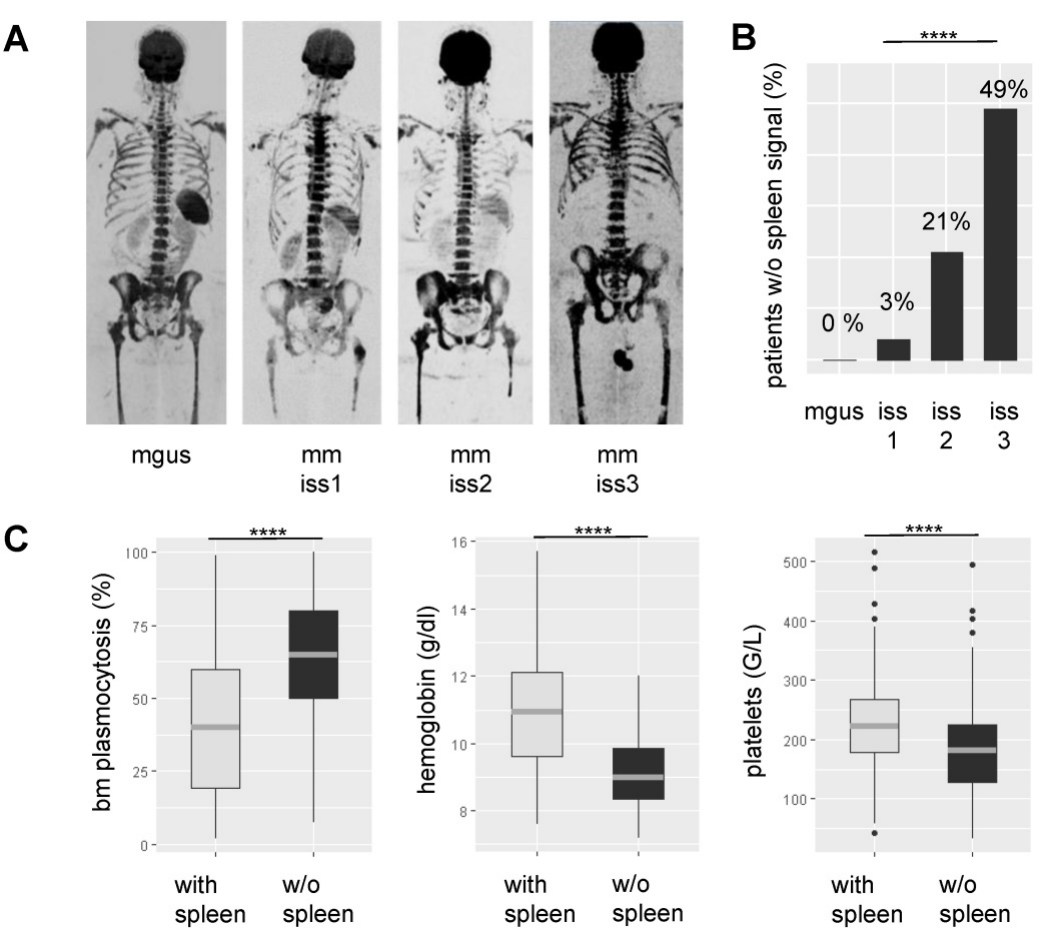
Association between spleen signal on DW-MRI, tumor load, and hematopoietic insufficiency. In A representative inverted greyscale b800 DW-MRI images for progressing stages of plasma cell disorders are shown. While the individual with monoclonal gammopathy of undetermined significance (MGUS) presents with a hyperintense spleen, the spleen signal gradually decreases and is absent in the multiple myeloma (MM) patient with International Staging System (ISS) 3. B shows the proportion of patients without spleen signal within progressing stages of the disease. In C the association between spleen signal and laboratory parameters is depicted. BM: bone marrow;  *P*< 0.0001
